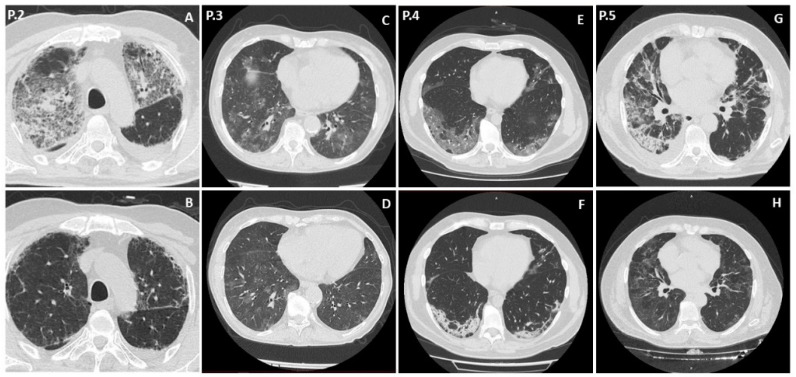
Radiological characteristics of the patients. Patient 2 (P.2): (A) HRCT at PJP diagnosis; (B) HRCT after CTX therapy, 21 days after PJP diagnosis. Patient 3 (P.3): (C) HRCT at PJP diagnosis; (D) HRCT after CTX therapy, 30 days after PJP diagnosis. Patient 4 (P.4): (E) HRCT at PJP diagnosis; (F) HRCT after CTX therapy, 20 days after PJP diagnosis. Patient 5 (P.5): (G) HRCT at PJP diagnosis; (H) HRCT after CTX therapy, 26 days after PJP diagnosis. HRCT = high-resolution CT-scan; PJP = Pneumocystis jirovecii pneumonia; CTX = trimethoprim-sulfamethoxazole.
